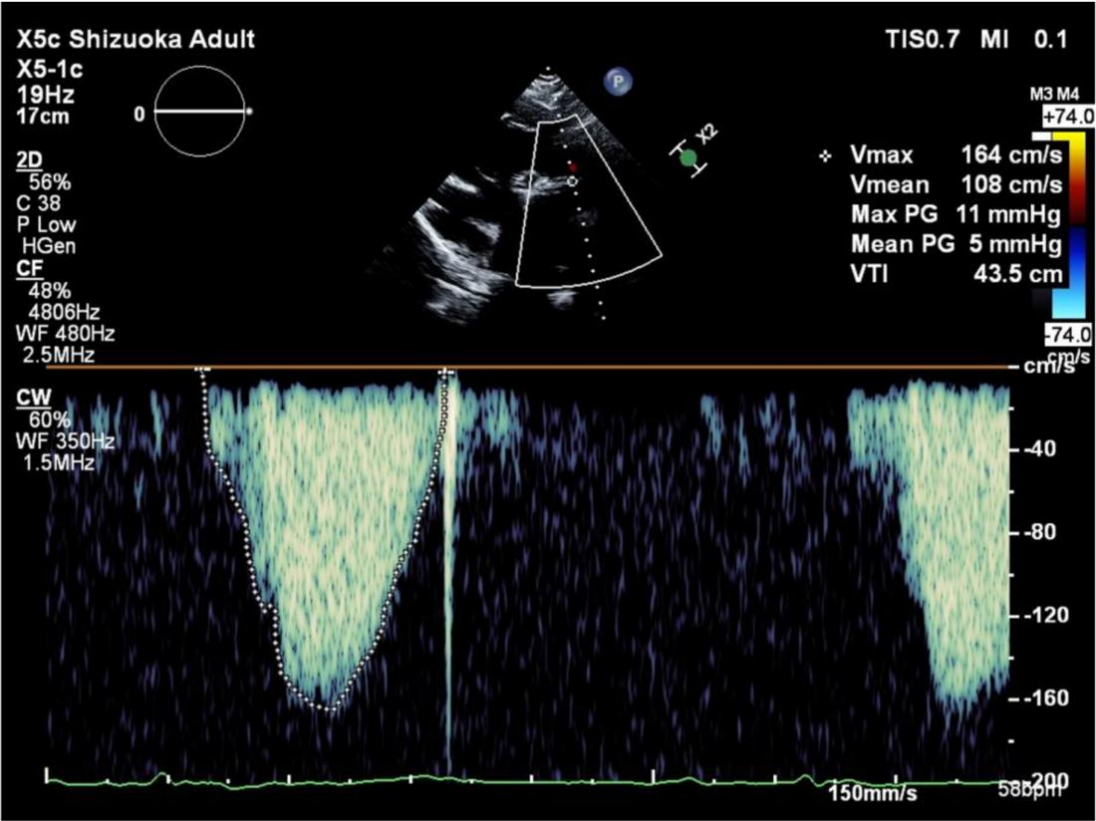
Transthoracic echocardiography on postoperative Day 5 confirming good mobility of the implanted valve and improvement of pulmonary stenosis (PS) and pulmonary regurgitation (PR), with no evidence of end-diastolic forward flow.
